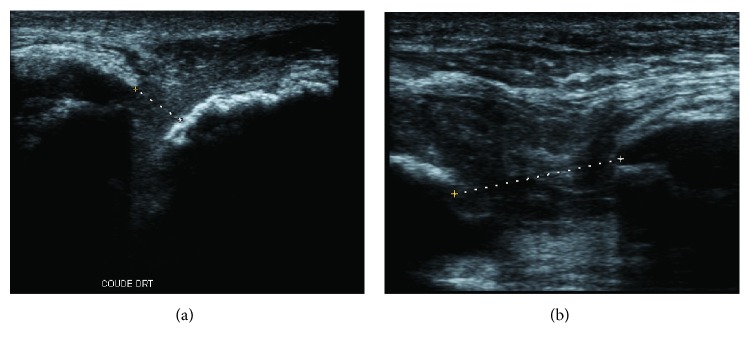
Ultrasound showing the tendinous gap of the triceps, extending further upon joint flexion: (a) gap in extension and (b) gap in flexion.
